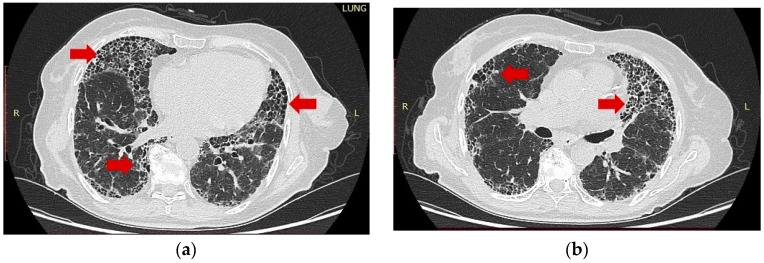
HR-CT scan of the same patient (cross-sections) showing bilateral moderately severe “honeycomb” fibrosis (red arrows) in an “usual interstitial pneumonia” pattern: (a) predominantly peripheral interstitial changes; (b) basal subpleural disposition of fibrosis thickened septa.
